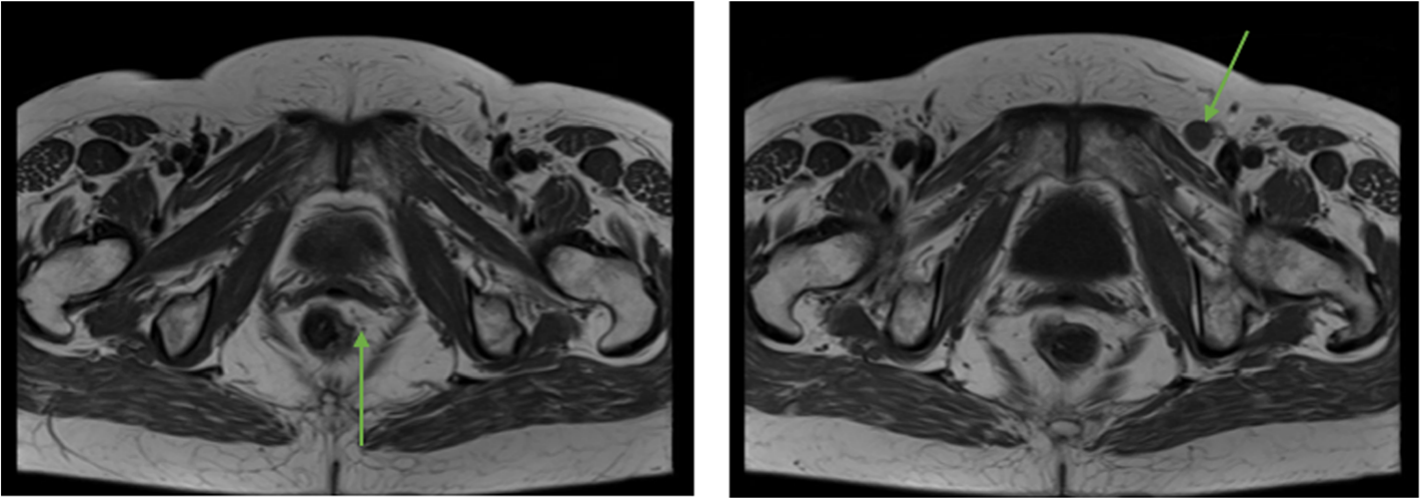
MRI axial images showcasing the initial 2014 tumor at the anorectal junction on the left lateral border (6 × 6 mm) and the left inguinal lymph node (1.2 × 1.2 cm)
